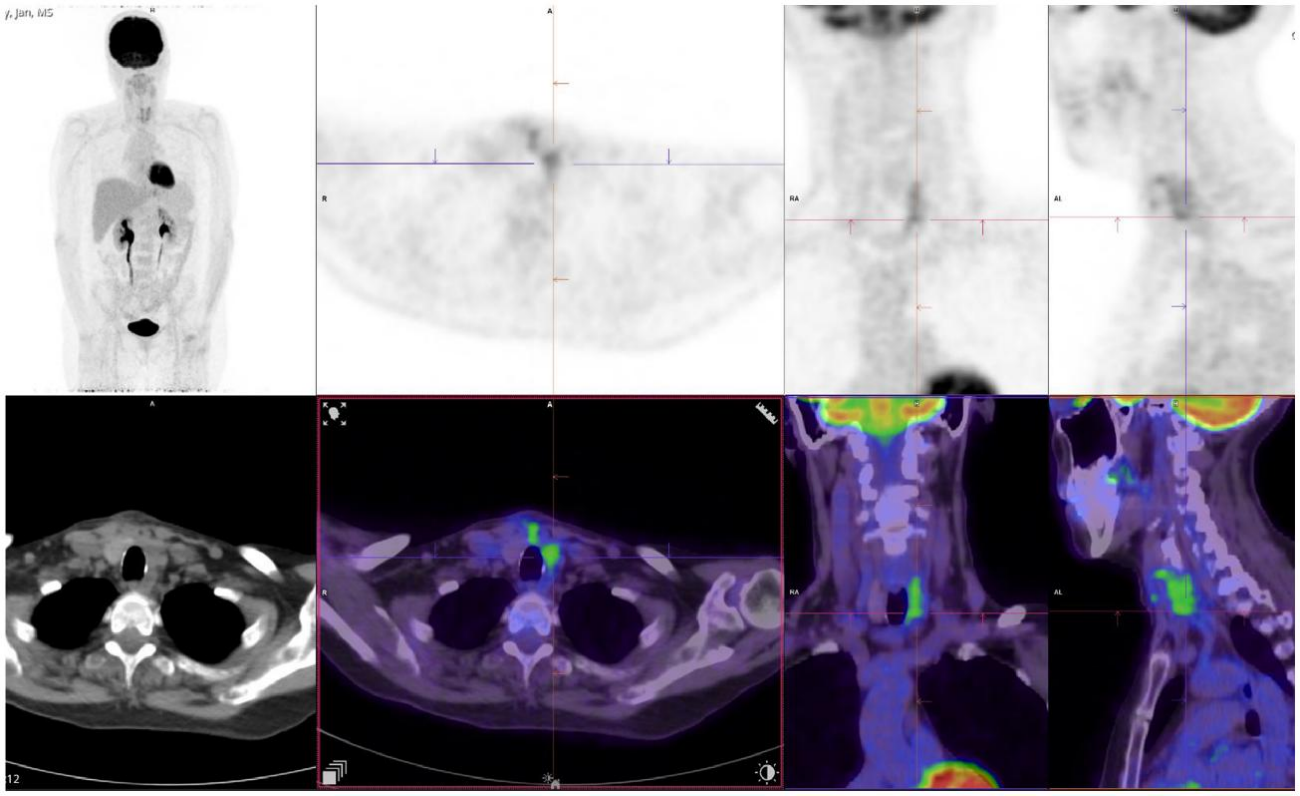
FDG PET-CT performed 1 month following surgical resection of left inferior parathyroid adenoma (February 2022). There was moderate metabolic activity in the left thyroid bed, isthmus, and cervical pretracheal region, and subcentimeter left supraclavicular fossa lymph node, which may be reactive to recent surgery; however, residual disease and nodal metastatic disease could not be excluded. There was no evidence of metabolically active distant metastatic disease. The patient was referred for radiotherapy to the surgical bed following review of images in a multidisciplinary meeting.
